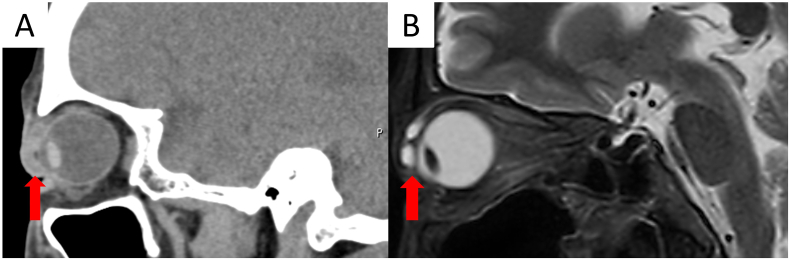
Imaging of the right eye. A. Orbital computed tomography showing a 17 × 15 × 8-mm mass that is slightly denser than the skin. B. Orbital T2-weighted magnetic resonance imaging showing two hyperintense and smooth-surfaced masses measuring 5 mm and 6 mm.
